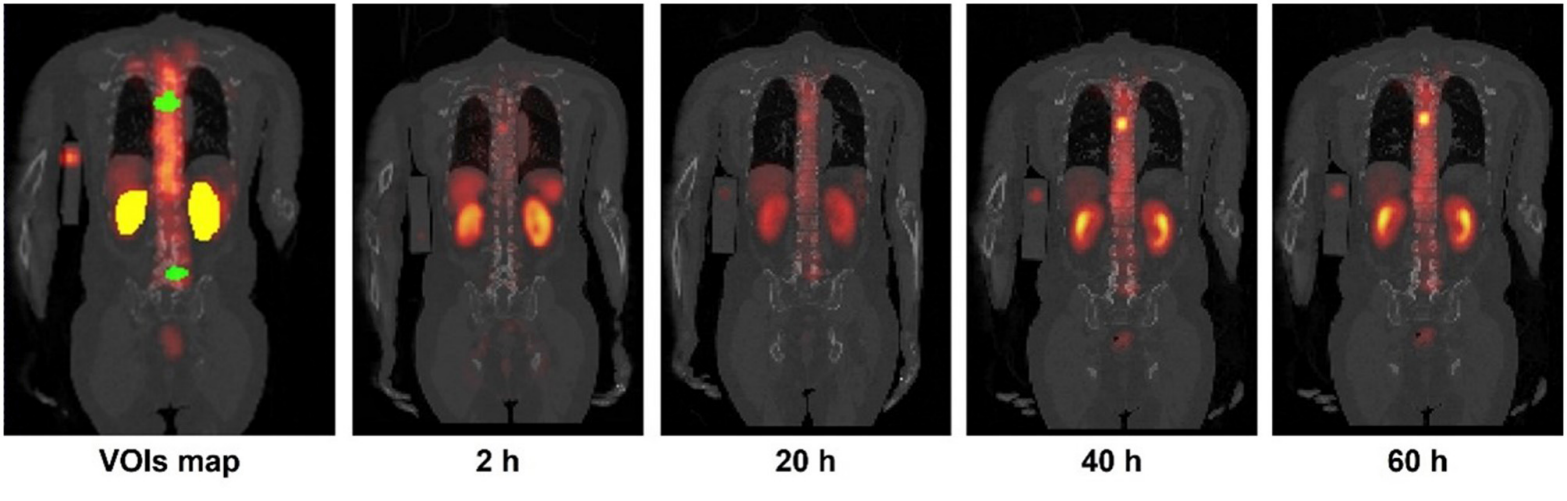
Sample kidneys (yellow) and tumor (green) maps delineated on CT and SPECT fusion images. Sample 4TP sequential SPECT/CT images acquired after Lu-177-PSMA administration are also shown.
